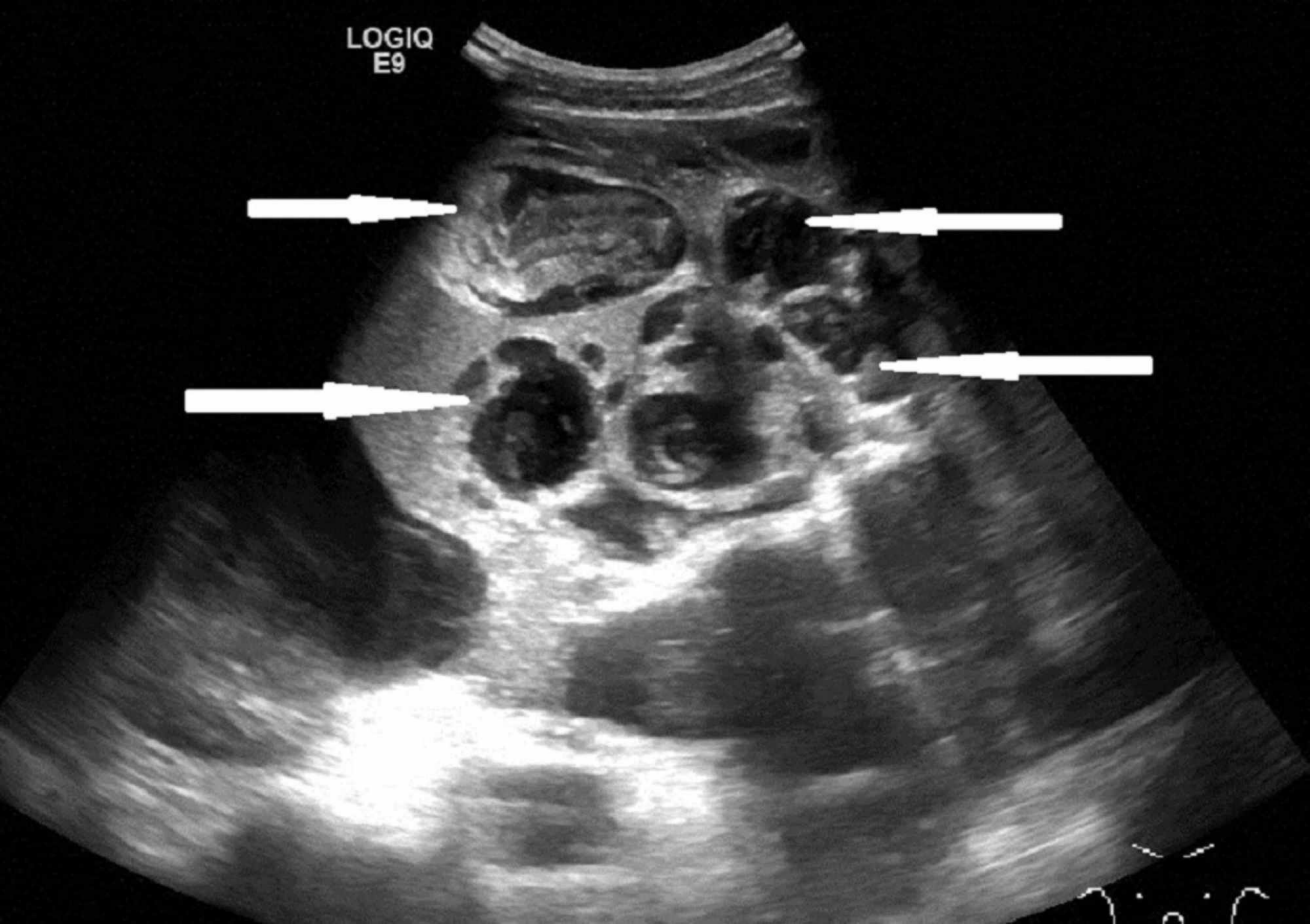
Ultrasound of abdomen showing multiloculated ascites with extensive internal debris (arrows)
